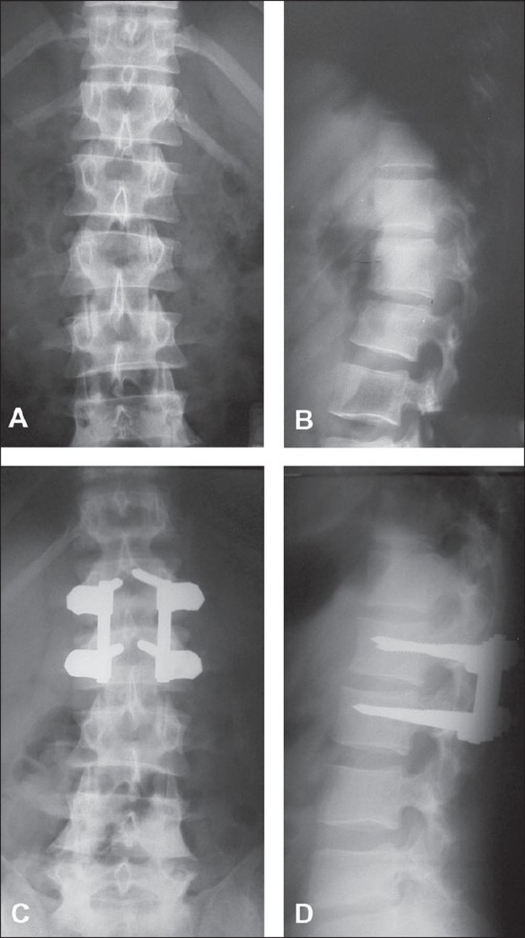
Preoperative X-rays of dorsolumbar spine AP and lateral (A & B) show a type B lumbar vertebral fracture. The patient was treated with a posterior monosegmental fixation- The immediate post operative X-ray AP and lateral (C & D) shows the posterior monosegmental fixation
